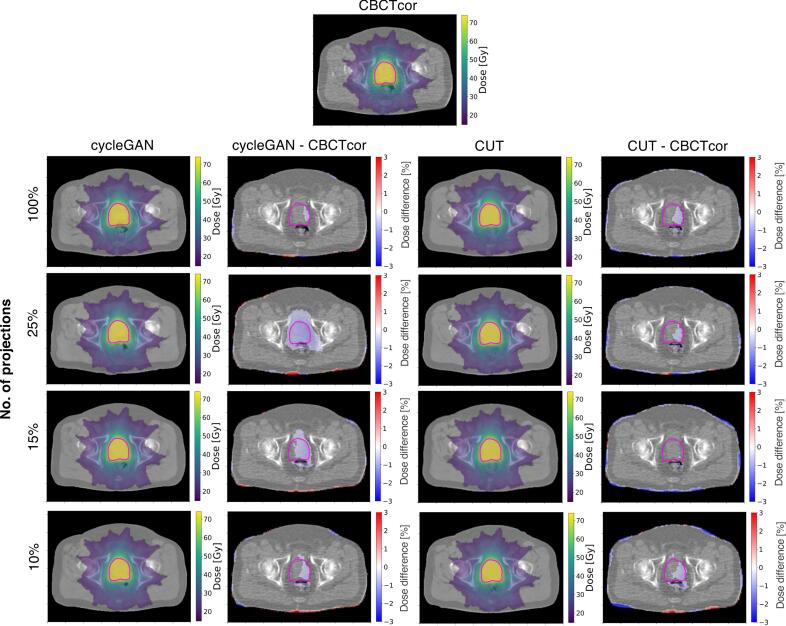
VMAT dose distributions of an exemplary patient. Dose distributions optimized on CBCT_cor_ and recalculated on sCTs at difference dose reduction levels, and their corresponding differences for cycleGAN and CUT. The PTV is shown in magenta. Dose differences below 0.4% are not shown for better visualization.
